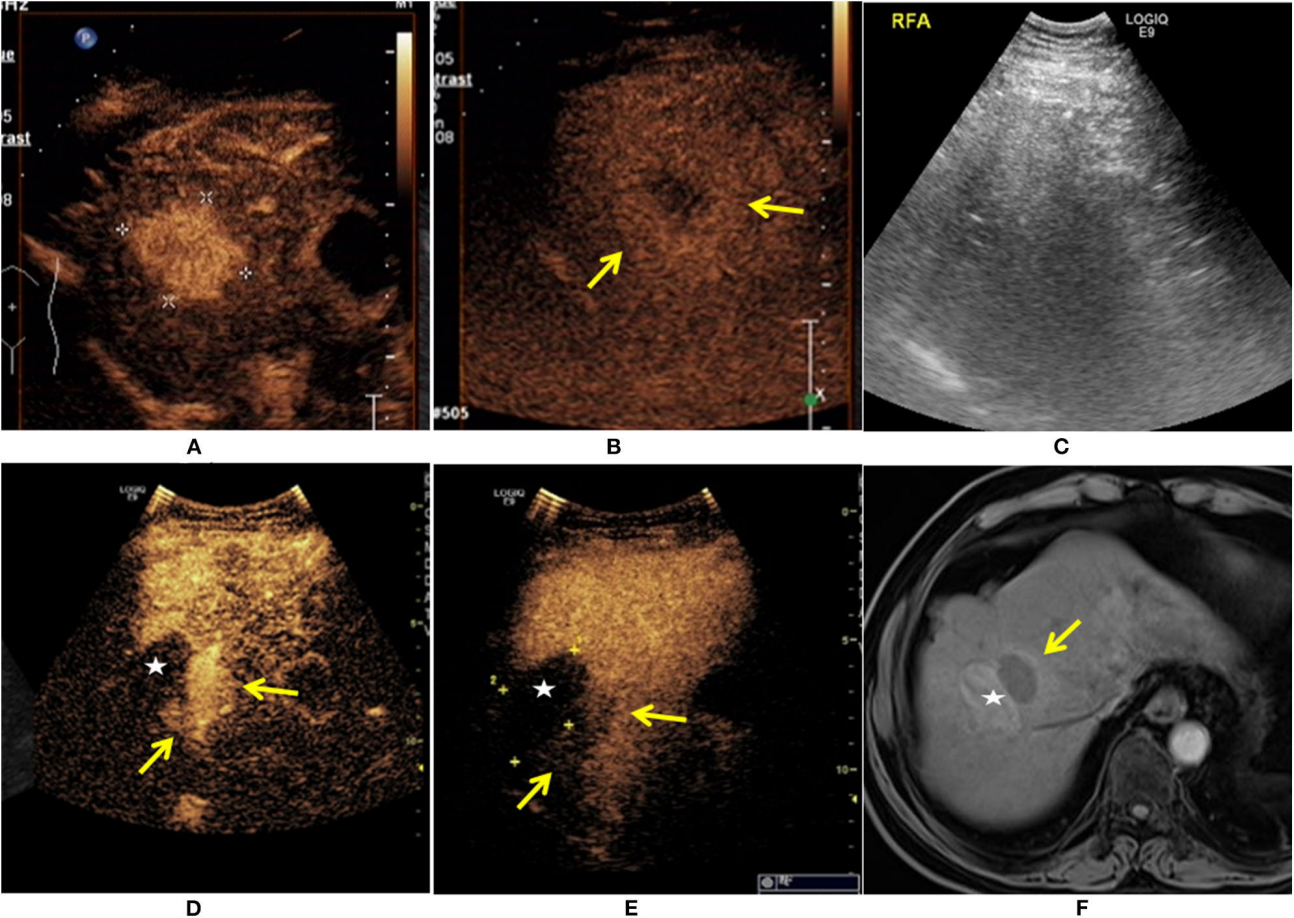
A 65-year-old man was diagnosed with HCC. The patient had a 30-year history of hepatitis B. The contrast-enhanced ultrasound (CEUS) showed a single lesion measuring 4.1 × 3.6 cm in size (+) enhanced at arterial phase (A), and the mild washout in lesion was found at the late phase (
